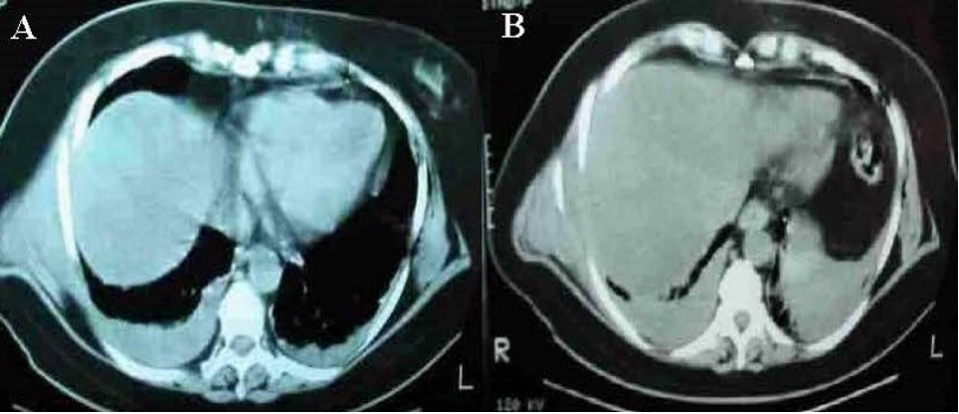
Thoracic CT with bilateral hemothorax Thoracic CT revealed hemothorax bilaterally, but especially on the right, hypoventilation of lower lung fields, left subcutaneous emphysema, rib fractures (6th, 7th, 8th right ribs / 7th, 8th, 9th left ribs), resulting in flail chest. In addition, a large soft tissue hematoma associated to the fractures of the lower thoracic ribs was detected (A.) Abdominal CT revealed herniation of hepatic parenchyma through the thoracic wall with concomitant hematoma (B.).
